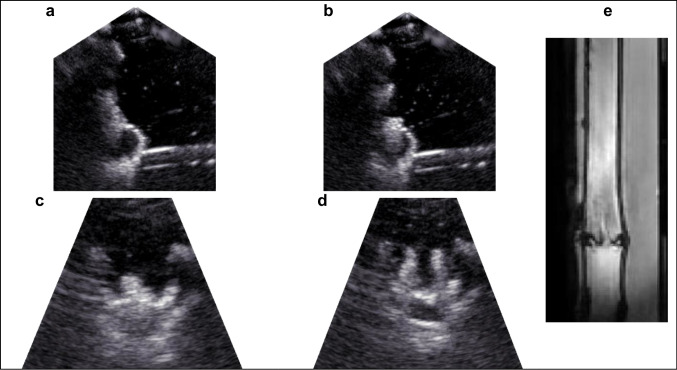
Images of a valve in closed (a and c) and open (b and d) conformation in US and in MRI (e)
